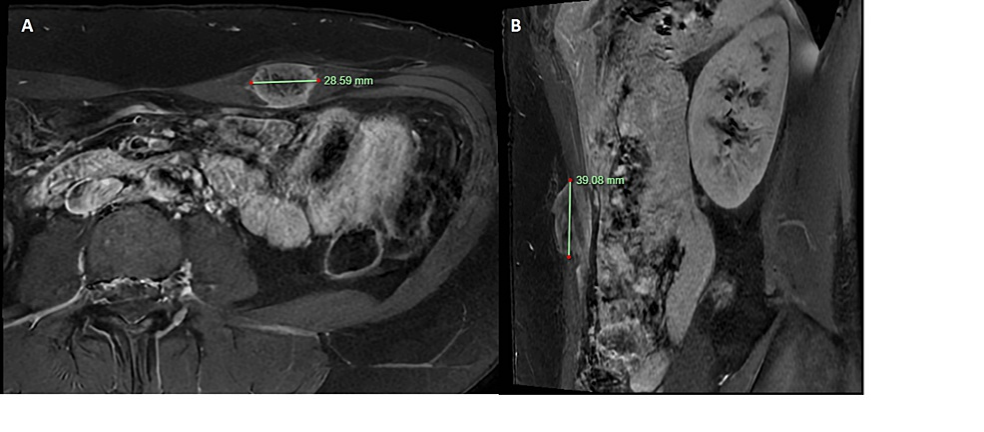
Magnetic resonance image of the patient's abdominal wall showing T1 phase post-contrast in (A) axial and (B) sagittal views. The tumour can be seen as a hypointense mass within the left rectus abdominis muscle with contrast rim enhancement.
